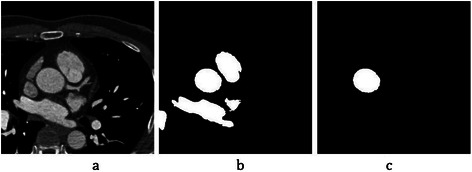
Aorta segmentation in initial slices. (a) A selected slice from the initial CTA slices. (b) Extraction of region 4 based on Hounsfield Unit (*HU*) measurement and also pre-processing steps for removing small objects. (c) The result of aorta segmentation by applying Hough circle transform and performing some post-processing steps.
